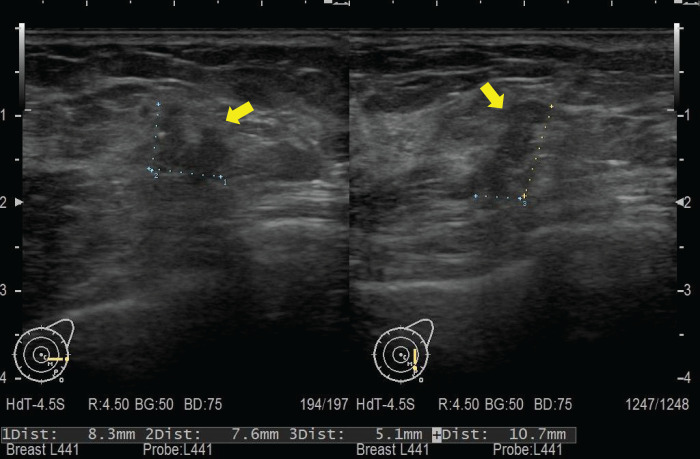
Ultrasonographic findings. Ultrasonography revealed an irregular mass in the outer region of the left breast, measuring 0.8 × 0.5 × 1.0 cm (yellow arrow). The nipple–tumor distance was 4.6 cm.
